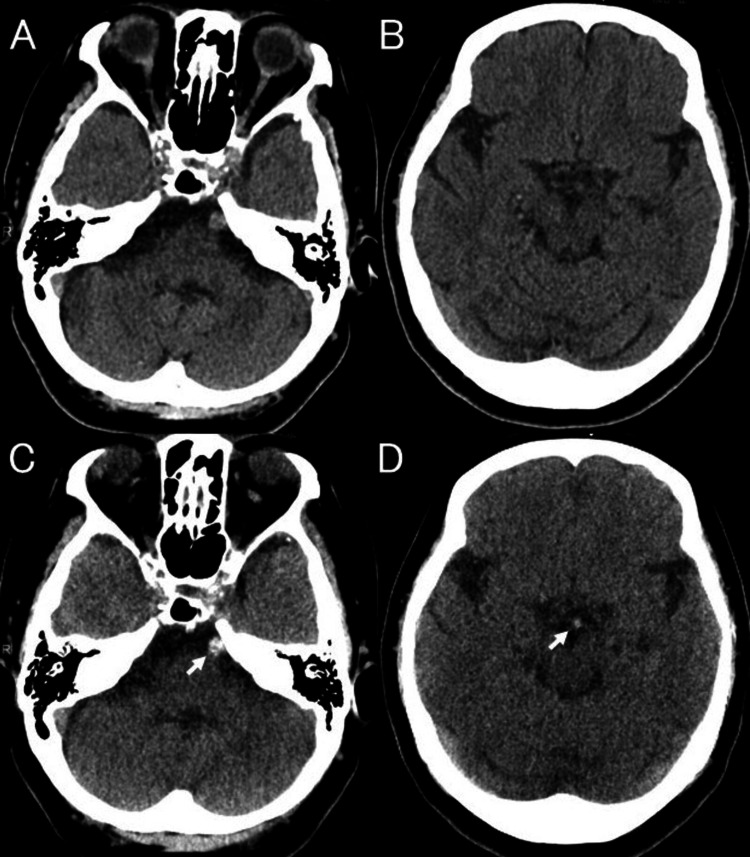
(A,B) Previous CT images compared with (C,D) noncontrast-enhanced CT obtained three hours after symptom onset reveal an HDBA sign (arrows) CT: computed tomography; HDBA: hyperdense basilar artery
